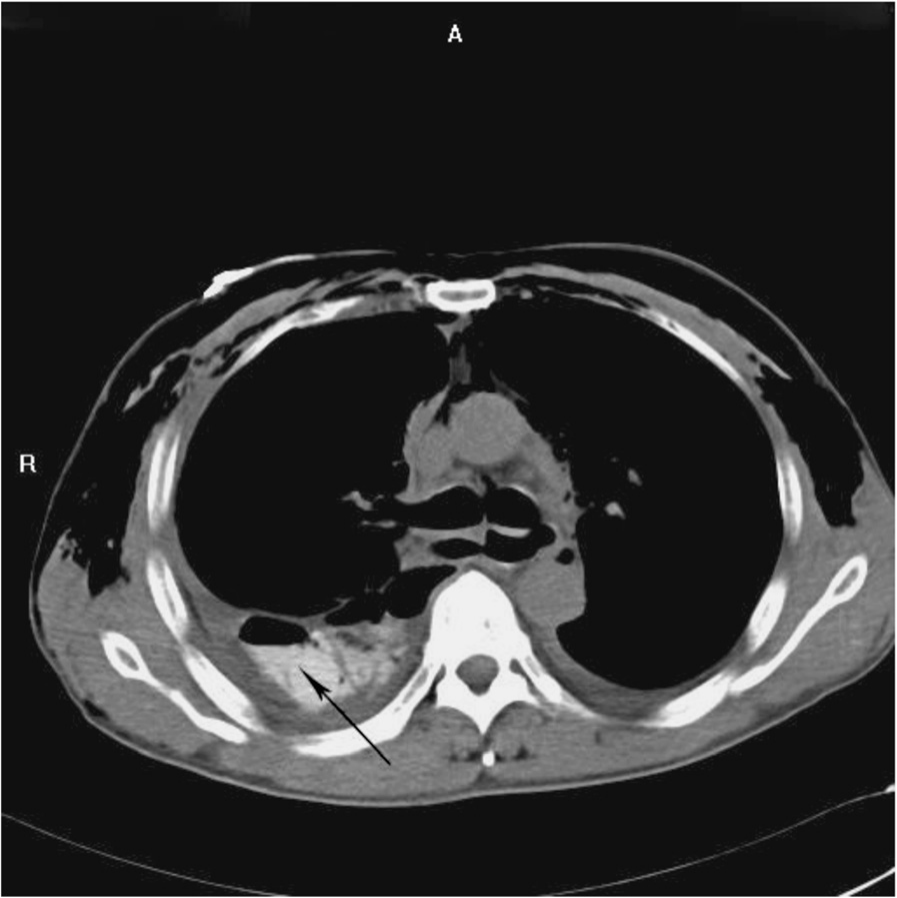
Chest computed tomography scan and barium swallow examination indicated esophageal rupture. Arrow: barium entered into thoracic cavity through the ruptured esophagus. A: anterior. R: right.
